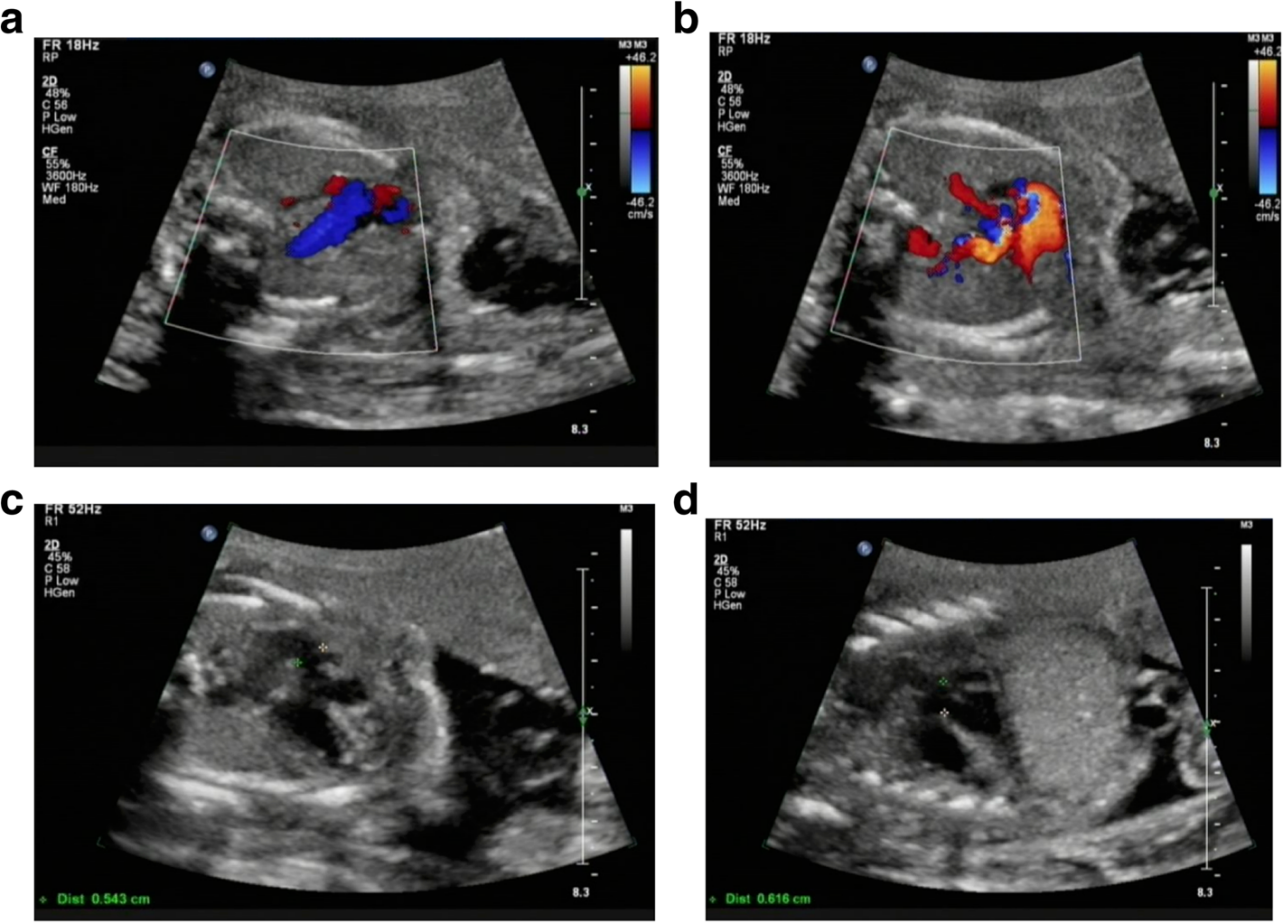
fetal echocardiography of the second fetus. a three vessels views in fetal echocardiography; b persistent trunk; c and (d) ventricular septal defect
